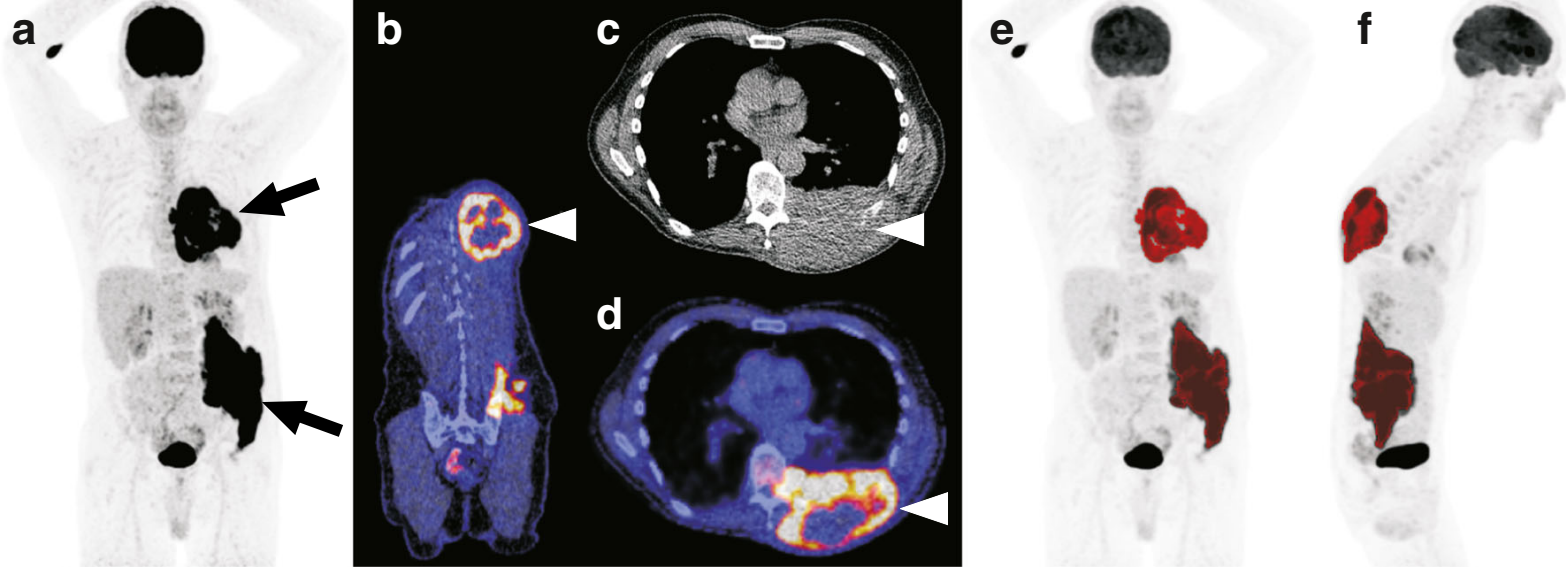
Visual assessment of necrosis and semiquantitative ^18^F-FDG PET review process. a A 65-year-old man with diffuse large B cell lymphoma (DLBCL) and tumour masses in the left dorsal chest wall and left pelvis, as shown on the coronal maximum intensity projection (MIP) ^18^F-FDG PET image (arrows). Coronal fused ^18^F-FDG PET/CT (b), axial CT (c) and axial fused ^18^F-FDG PET/CT (d) show the tumour mass with photopenic areas (arrow heads), in keeping with tumour necrosis. Coronal and sagittal MIP ^18^F-FDG PET images (e and f) show tumour segmentation (marked in red colour) for the calculation of metabolically active tumour volume (MATV), total lesion glycolysis (TLG), maximum standard uptake value (SUV_max_), and single highest SUV_max_
